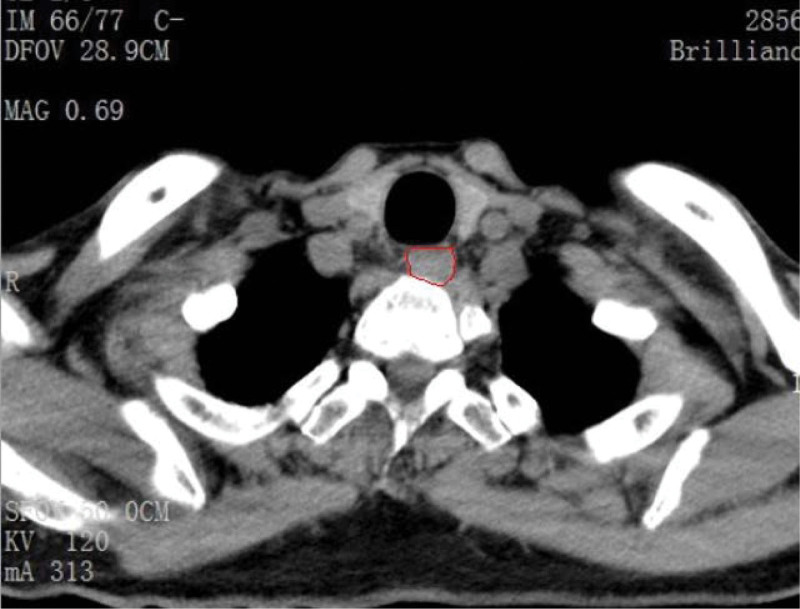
Esophageal area was measured in the level of T1 using CT axial plane images with Software of Digimizer Image Analysis. CT = computed tomography.
